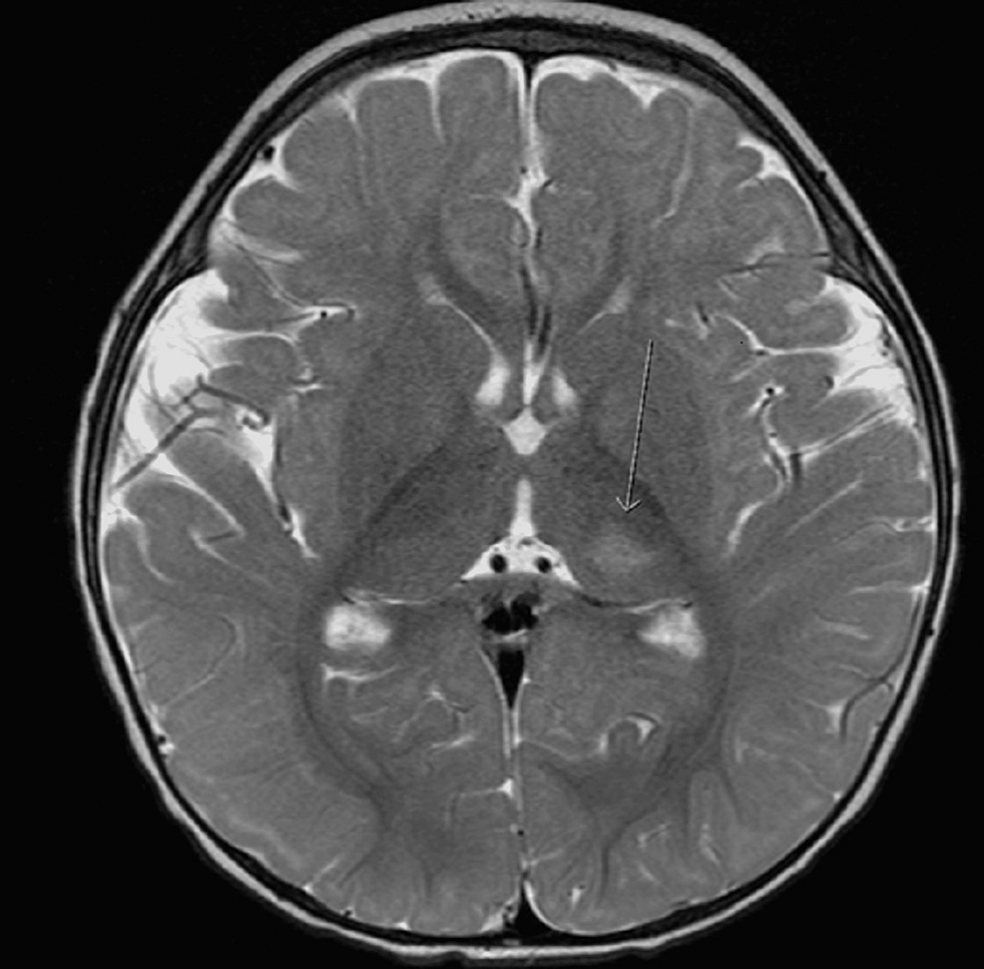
T2 FLAIR post-contrast MRI brain imaging showing high T2 signal in left thalamus.
